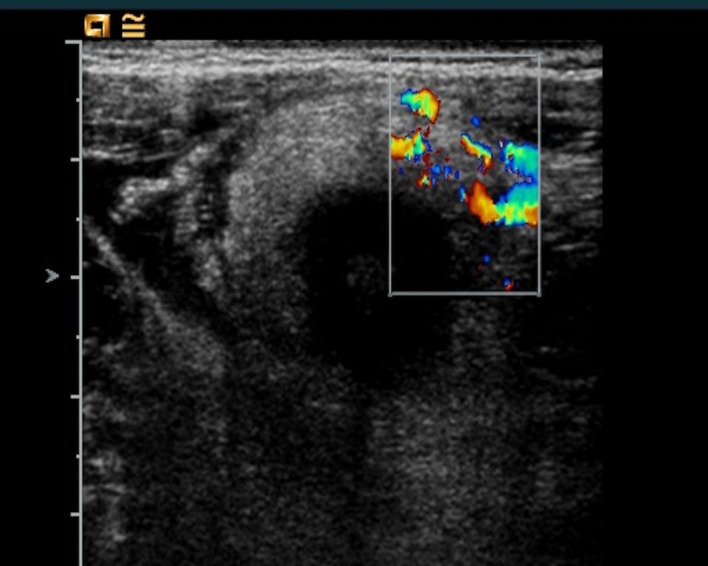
Figure 1:Abdominal ultrasound image of ileocecal wall thickening (3-6 mm) with increased signal in the vascular color-doppler and hyperplasia of the surrounding fatty tissue.
